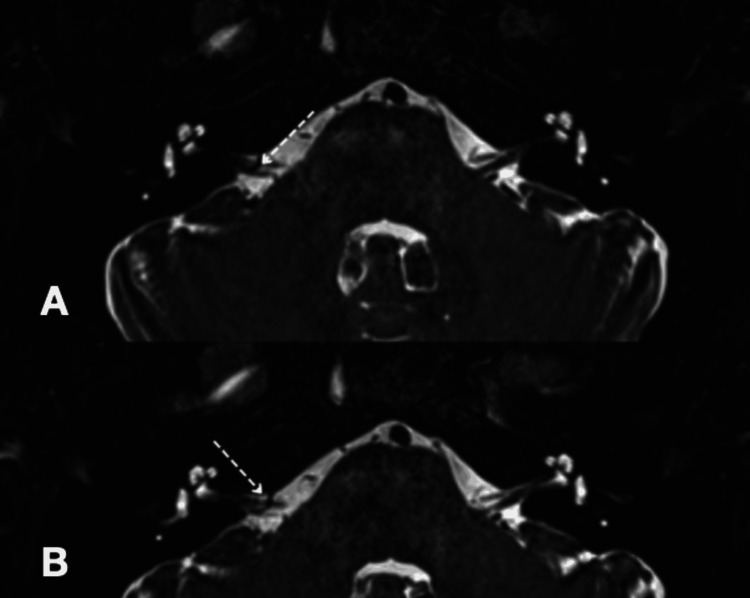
Magnetic resonance image analysis of Case 3 of constructive interference in steady state (CISS) sequence (A and B) showing a vascular loop at the right cerebellopontine angle causing mild compression and displacement of the seventh and eighth cranial nerve complex along the inferior aspect (dotted arrow).
